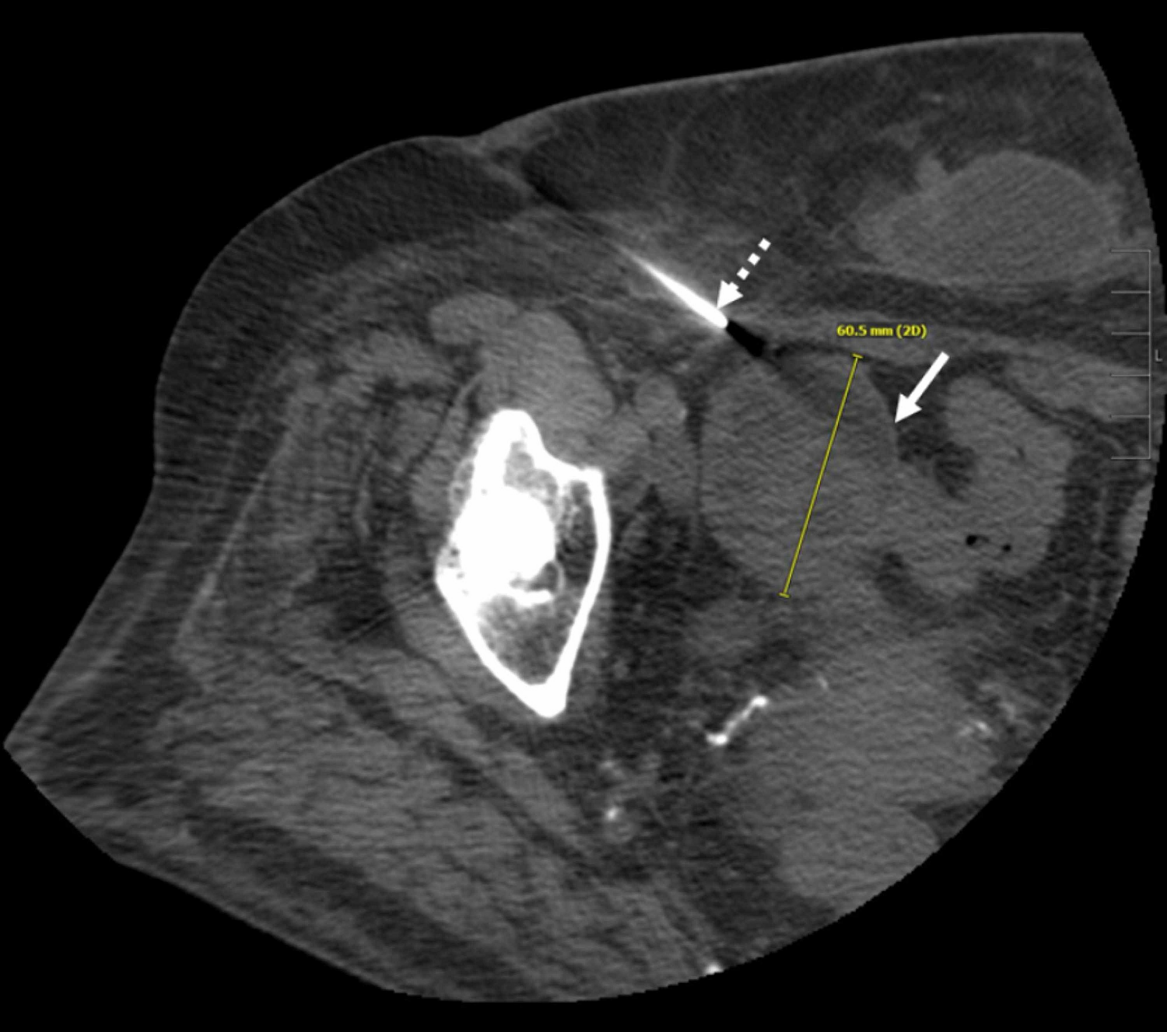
CT scan of the pelvis demonstrating percutaneous biopsy of the right ovarian mass Axial non-contrast CT obtained at time of biopsy six weeks prior to ablation demonstrates a well-circumscribed right adnexal mass (white arrow) measuring 6.0 cm in greatest dimension. Biopsy needle is seen from a right lateral approach (dashed arrow).
